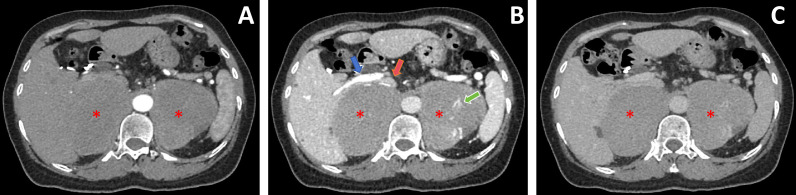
Dynamic contrast-enhanced CT images of bilateral adrenal masses. (A) Arterial phase: Large soft-tissue masses (asterisks) are present in the bilateral adrenal regions. (B) Venous phase: Large soft-tissue masses (asterisks) are present in the bilateral adrenal regions. This phase clearly demonstrates vessels traversing within and around the masses (green arrows), with compression and anterior displacement of the inferior vena cava (red arrow) and right renal vein (blue arrow). (C) Delayed phase: Large soft-tissue masses (asterisks) are present in the bilateral adrenal regions.
